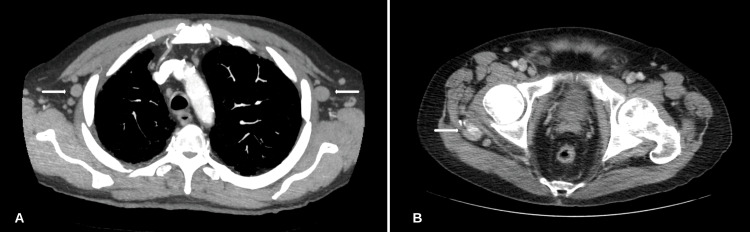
CT scan of the chest, abdomen, and pelvis. (A) Bilateral axillary lymphadenopathy. (B) A 1.0 cm rounded soft tissue nodule along the fascial plane between the right gluteus medius and maximus muscles is significant for metastatic disease. CT: computerized tomography
